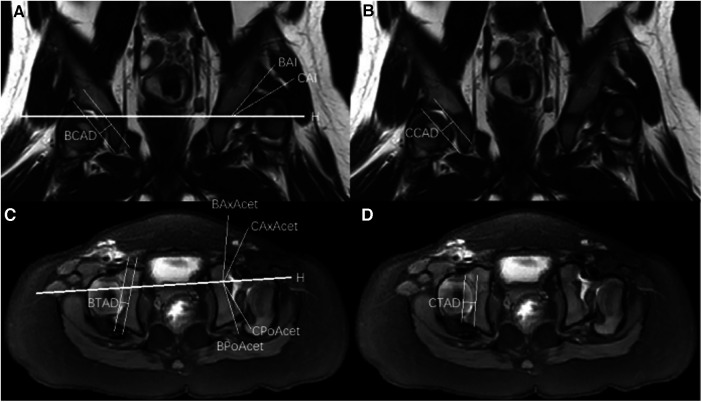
(A) Coronal T2-weighted magnetic resonance imaging demonstrating Hilgenreiner's line (H-H), boney acetabular index (BAI), cartilaginous acetabular index (CAI) and boney coronal acetabular depth (BCAD). (B) Coronal T2-weighted magnetic resonance imaging demonstrating cartilaginous coronal acetabular depth (CCAD). (C) Transverse T1-weighted magnetic resonance imaging demonstrating Boney axial acetabular angle (BAxAcet), cartilaginous axial acetabular angle (CAxAcet), boney posterior acetabular angle (BPoAcet), cartilaginous posterior acetabular angle (CPoAcet) and boney transverse acetabular depth (BTAD). (D) Transverse T1-weighted magnetic resonance imaging demonstrating cartilaginous transverse acetabular depth (CTAD).
